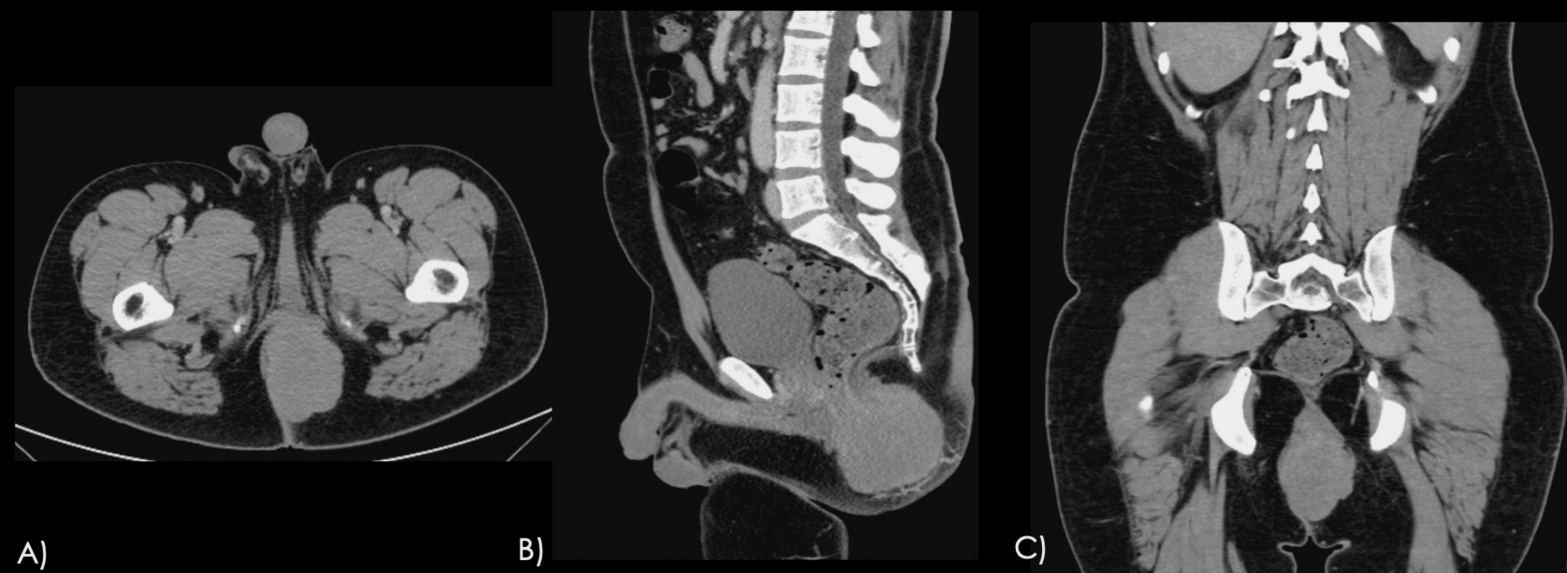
Baseline contrast-enhanced CT Baseline contrast-enhanced CT scan in the venous phase: (A) axial, (B) sagittal, and (C) coronal sections. A well-defined, lobulated mass with slight homogeneous contrast uptake, centered in the anal canal, causing rightward deformation of the gluteal fold. CT, computed tomography
